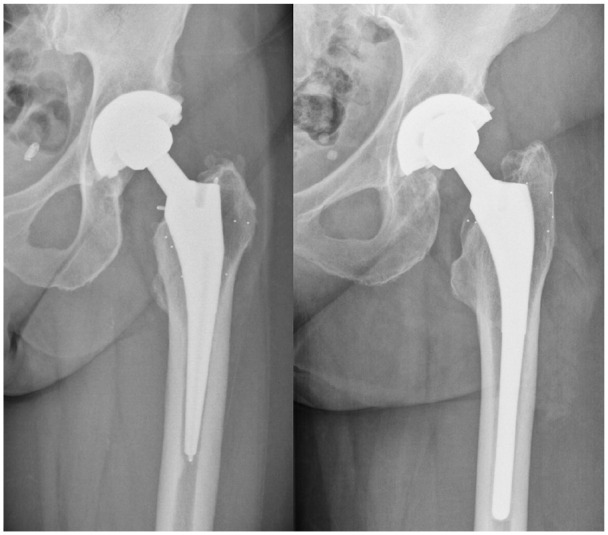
Radiographs of the Taperloc femoral stem on the left and the Mallory-Head femoral stem on the right.
